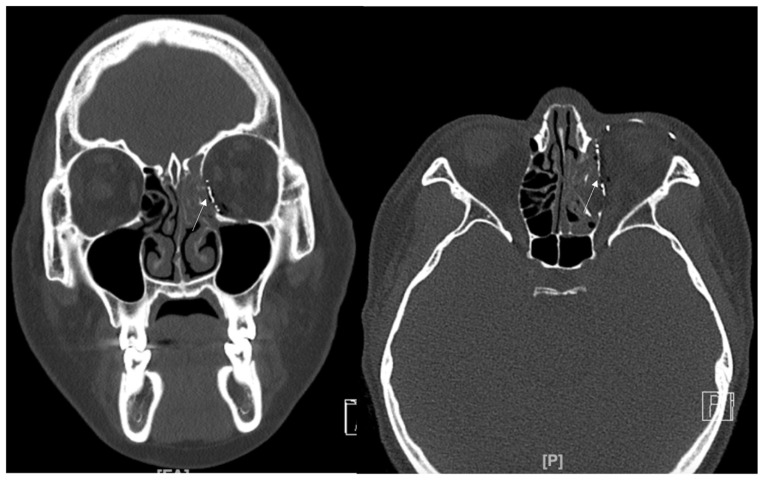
Case 3. Follow-up facial CT finding (coronal and axial view) of the same patient. The titanium-reinforced porous polyethylene (TR-PPE) plate was placed on the inferomedial orbital wall using a single screw at the inferior orbital rim. (White arrow).
